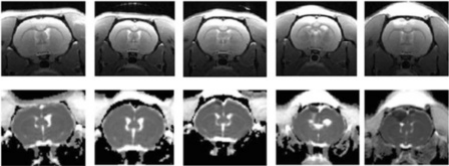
The T2 weighted (upper) and diffusion kurtosis (lower) images after 1 min ultrasound exposure are shown. The employed power from left to right is 1 W, 1.5 W, 2 W, 2.5 W and 3 W, respectively
